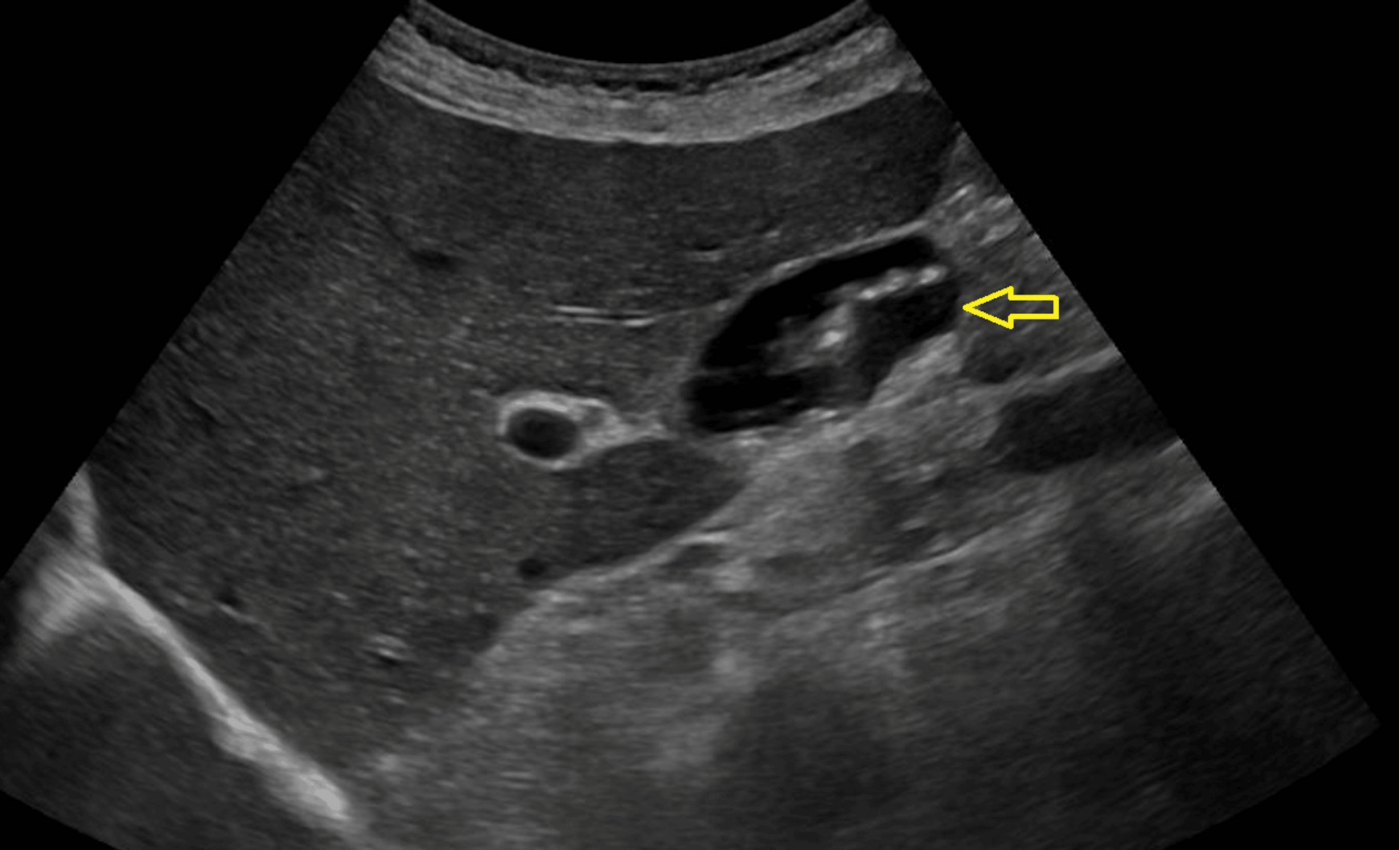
Ultrasound of the abdomen showing gall stone (arrow).
